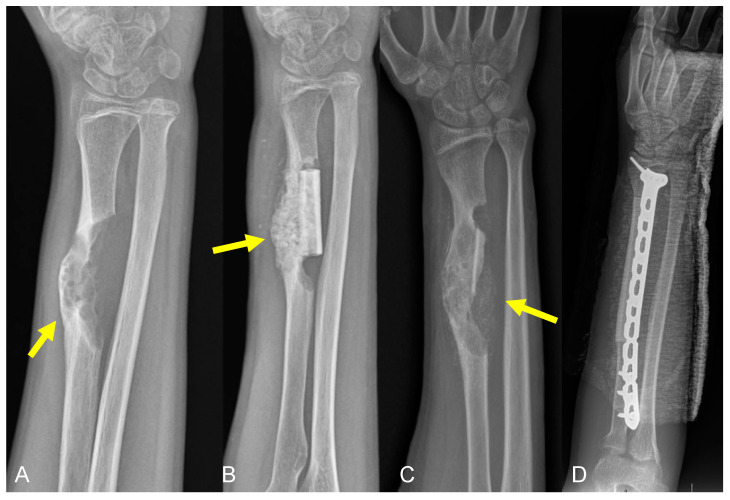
Plain radiographs of 16-year-old male with history of morphological variant of desmoplastic fibroma of the left radius (yellow arrows) treated with allograft reconstruction, now with tumor recurrence. Radiographs demonstrating (A) tumor recurrence after remote resection, (B) intralesional treatment of recurrence, and (C) second recurrence of tumor. (D) Post-operative radiograph showing repeat resection of tumor with new allograft reconstruction.
